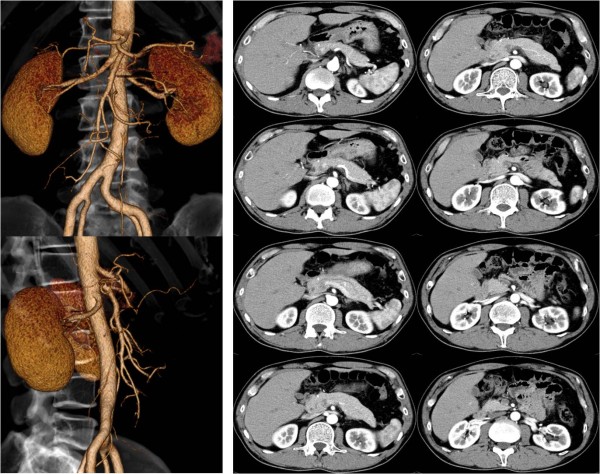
Follow-up computed tomography. The majority of changes continued to improve to almost normal over the next two-and-a-half years.
